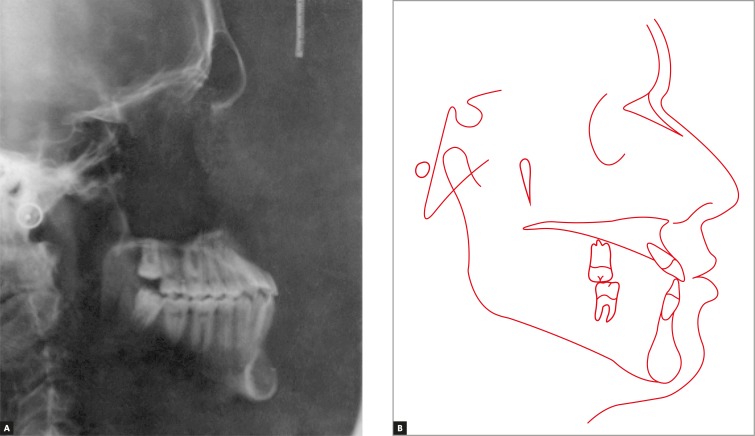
Final cephalometric profile radiograph (A) and cephalometric tracing (B).
